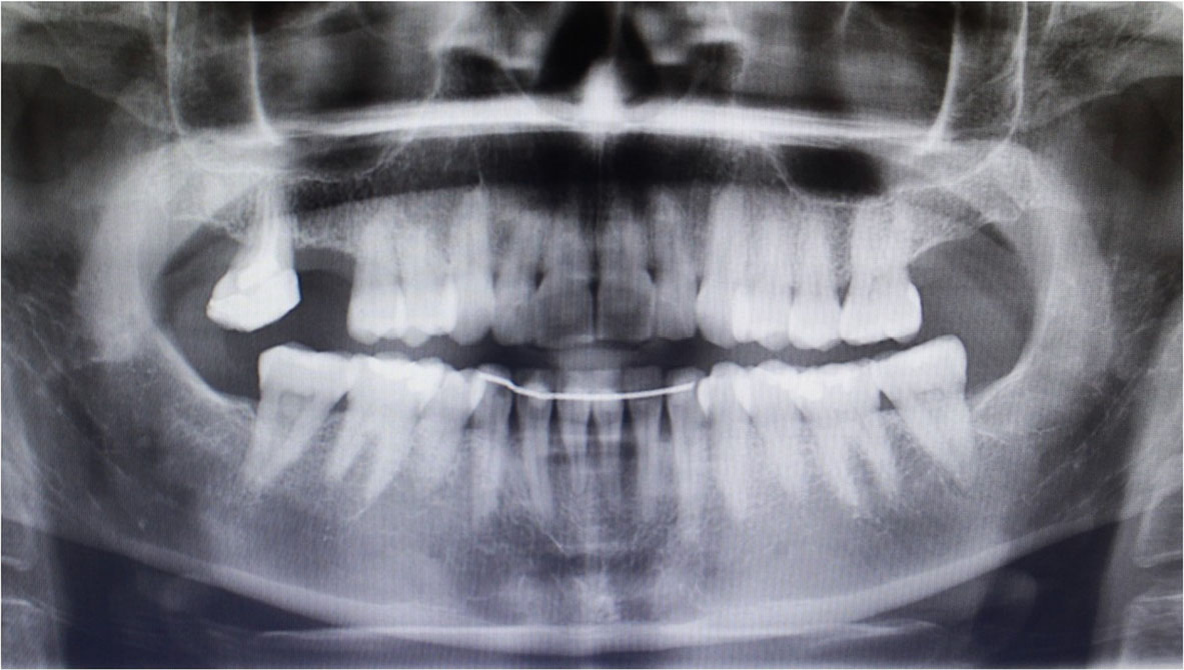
Diagnostic radiographic exploration previous to treatment
